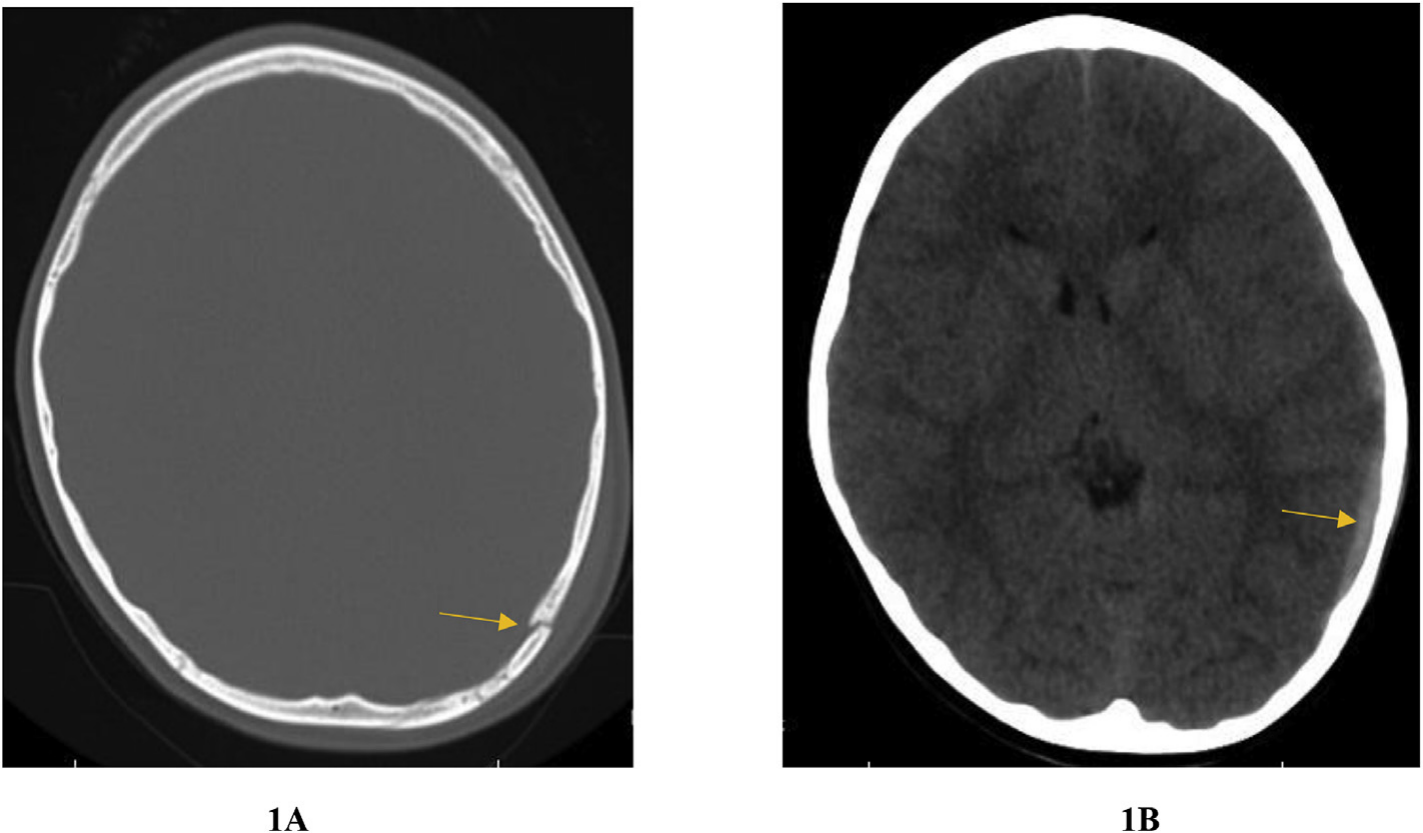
First CT scan showed a small, left-sided skull fracture (1A), and left-sided temporoparietal subdural (1B).
